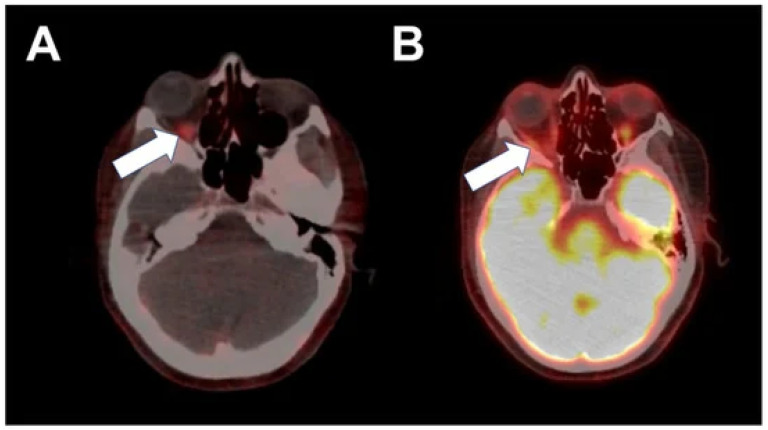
Abnormal intense [^18^F]FES activity in the right posterior orbit ((A), arrow) corresponding to the known right oculomotor nerve, right medial rectus, and superior aspect of the right inferior rectus. Normal physiologic [^18^F]FDG activity in the right extraocular muscles ((B), arrow) [31].
